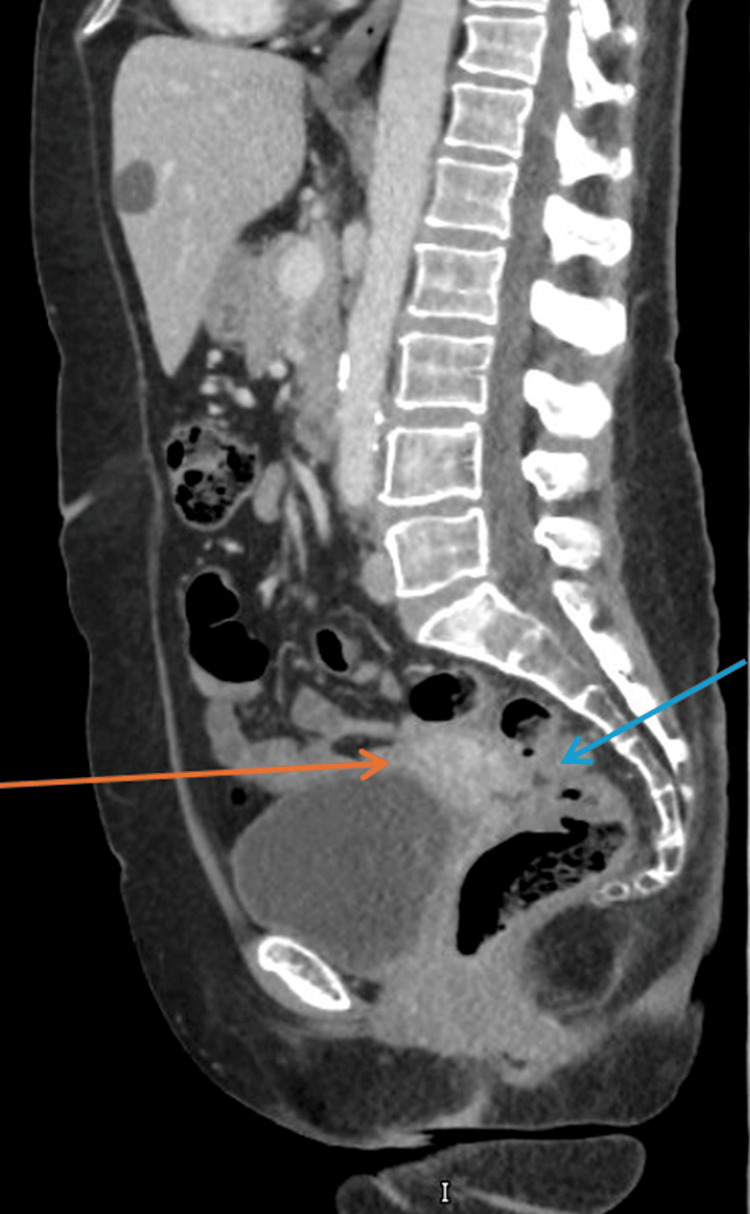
CT of thorax, abdomen and pelvis after four cycles of neoadjuvant chemotherapy, showing uterus has shrunk (orange arrow) and rectosigmoid appears to be inseparable from the uterus (blue arrow)
